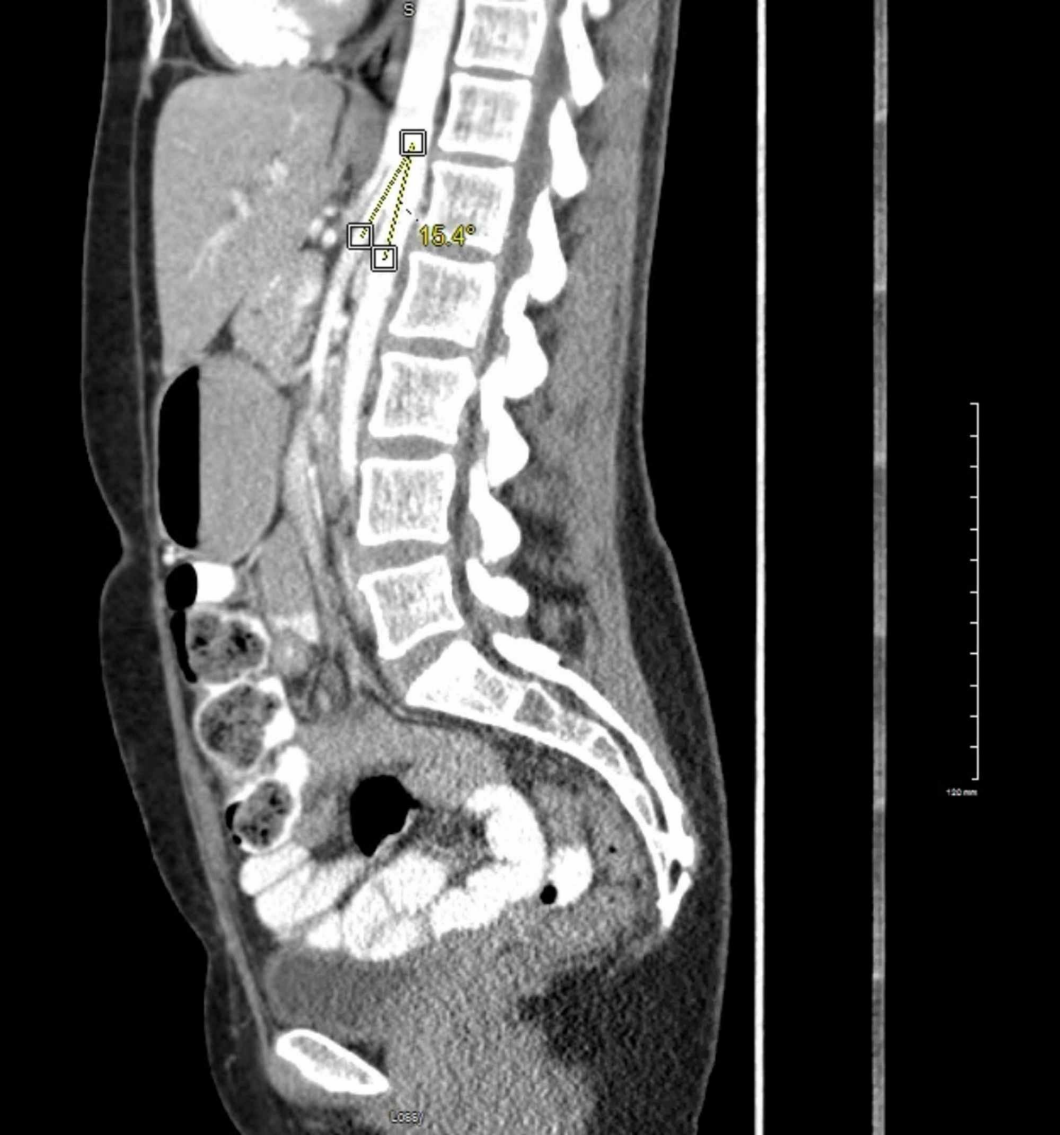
CT abdomen showing aorto-mesenteric angle of 15.4 degree
